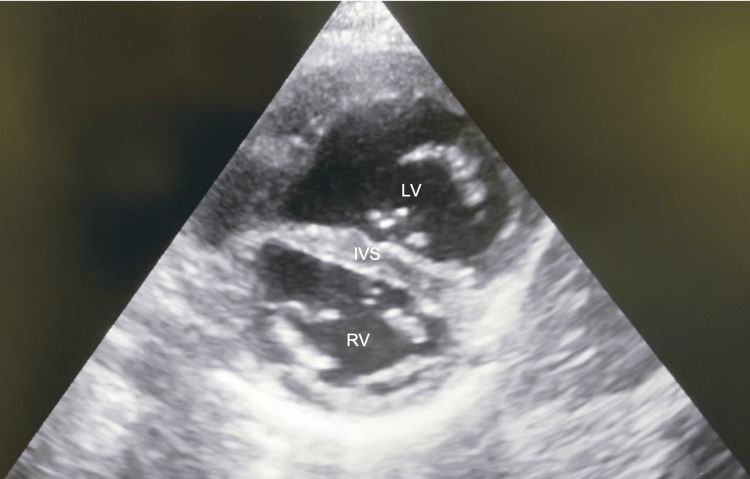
Trans-thoracic echo - parasternal short-axis view highlighting a straight intraventricular septum LV: Left ventricle; RV: right ventricle; IVS: intraventricular septum
